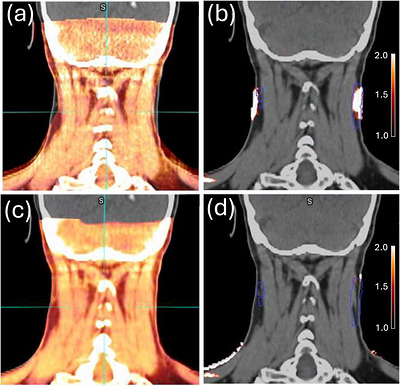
(a) Non‐pose corrected synthetic & replan CT fusion, (b) gamma index failing points (> 1) superimposed on CT for non‐pose corrected synthetic CT and replan CT dose distributions, (c) pose corrected synthetic & replan CT fusion and (d) gamma index failing points (> 1) for pose corrected synthetic CT and replan CT dose distributions.
